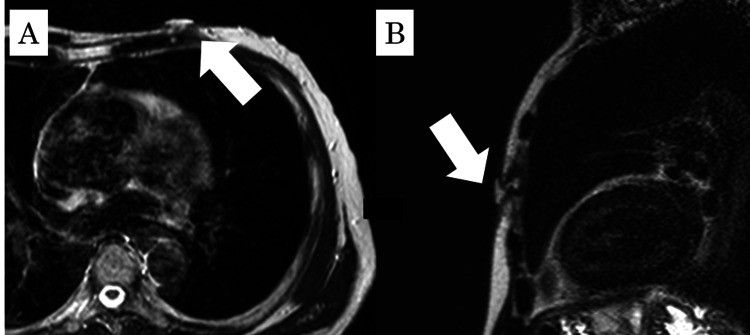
MRI images (T2-weighted sequence) (A) Axial view; (B) Sagittal view. A 20-mm lesion with high signal intensity was observed in the left anterior chest (arrows).
